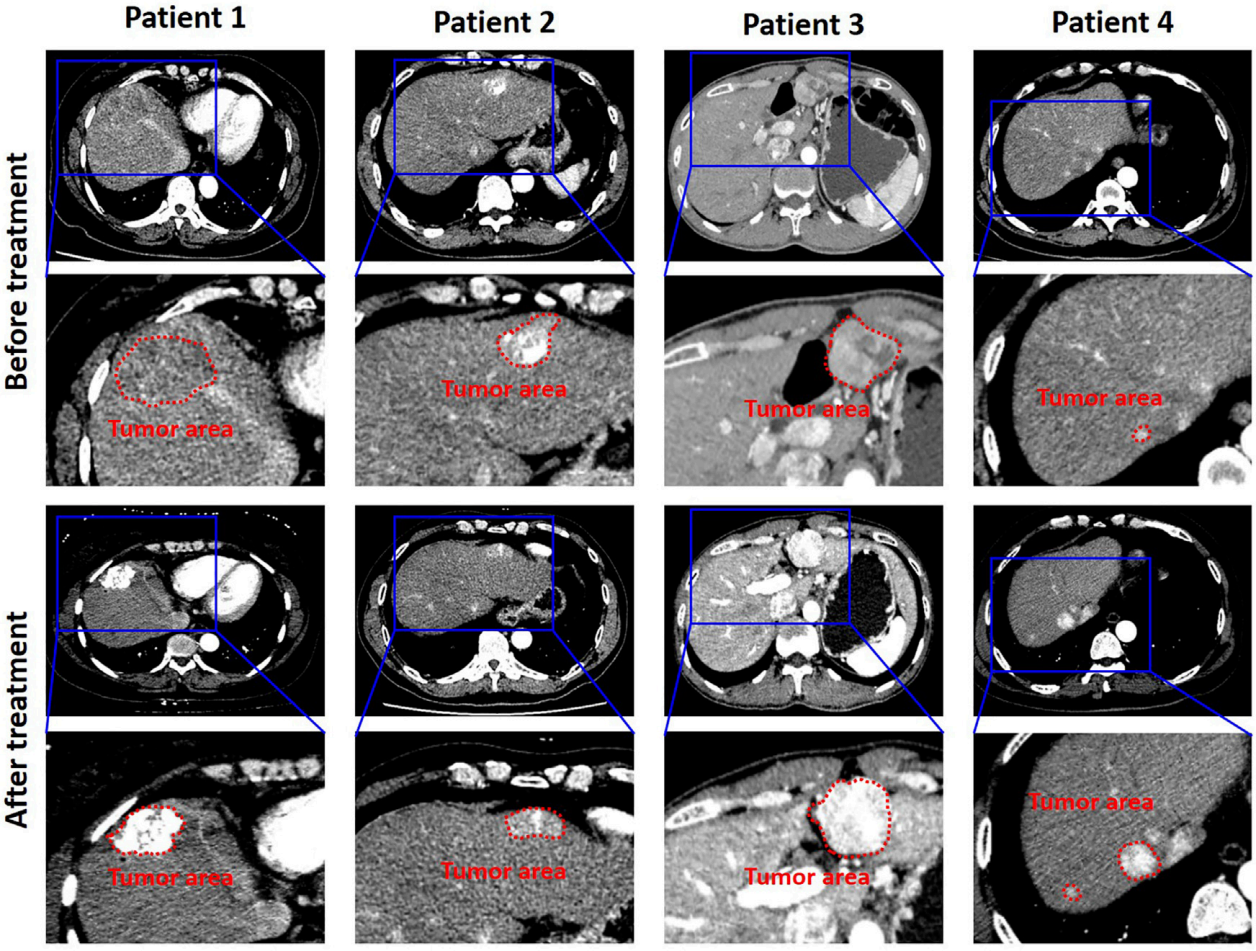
CT images were collected 7 days before treatment and 1 month after treatment, respectively. The measurement range was the longest diameter of the enhanced lesion in the tumor area. The tumor area of validation cohort with HCC of TACE therapy is shown in the red box.
